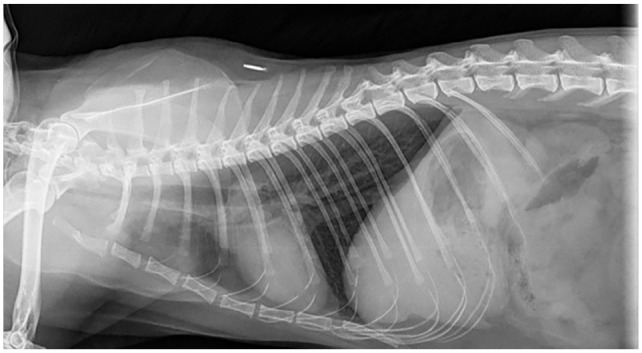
Right lateral thoracic radiograph showing sternal and tracheobronchial
